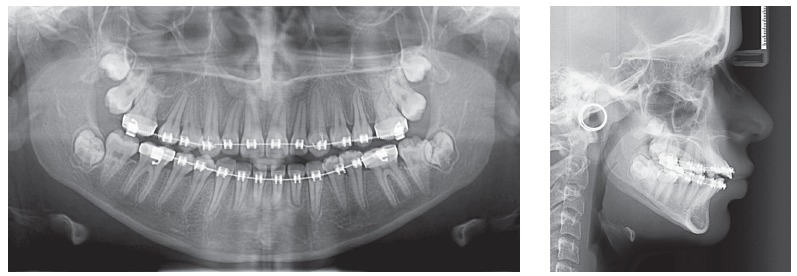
Panoramic and lateral radiographs showing the orthodontic appliance used in previous treatment.
